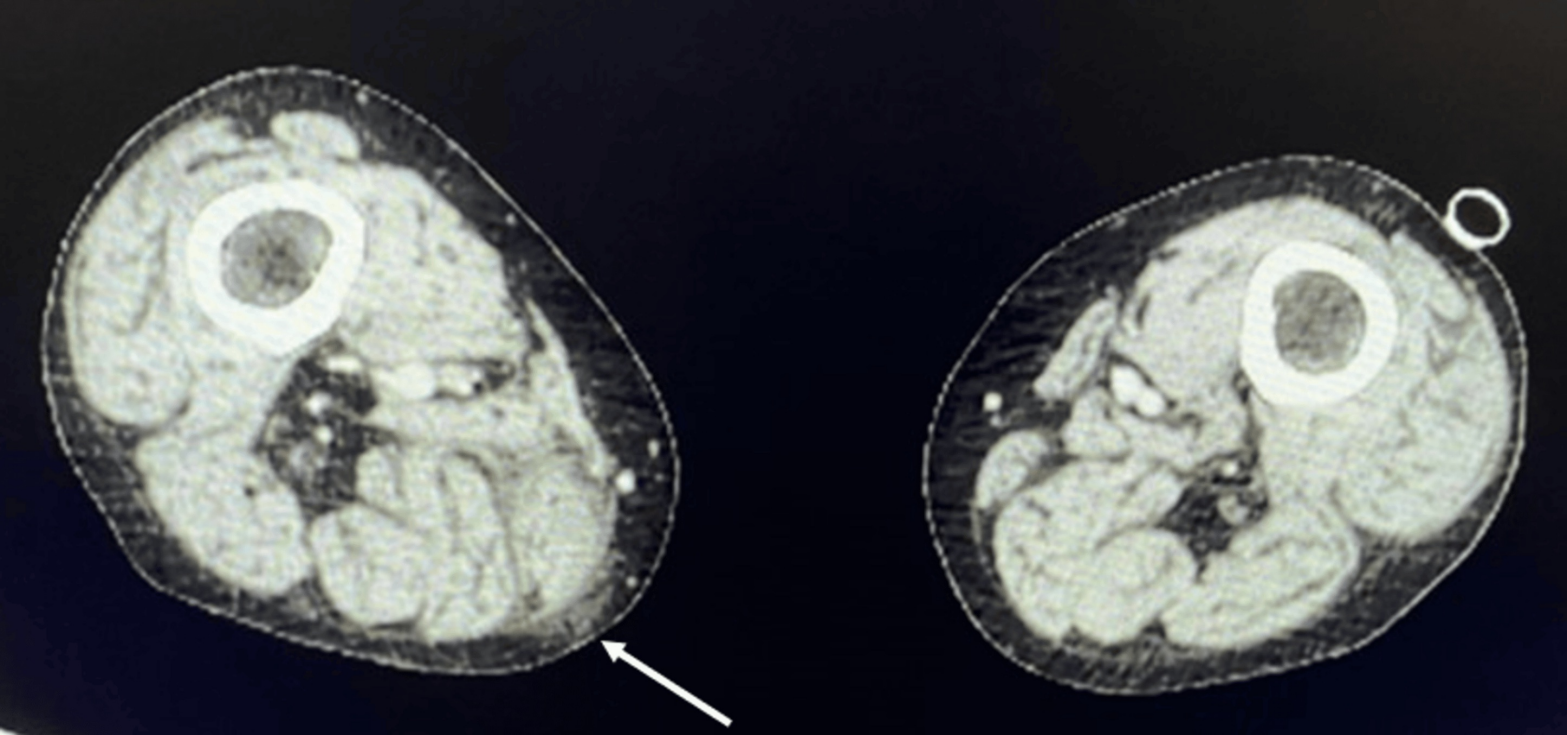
Contrast-enhanced CT at initial presentation There is edema around the gracilis muscle on the medial side of the right thigh (white arrow). CT: computed tomography
